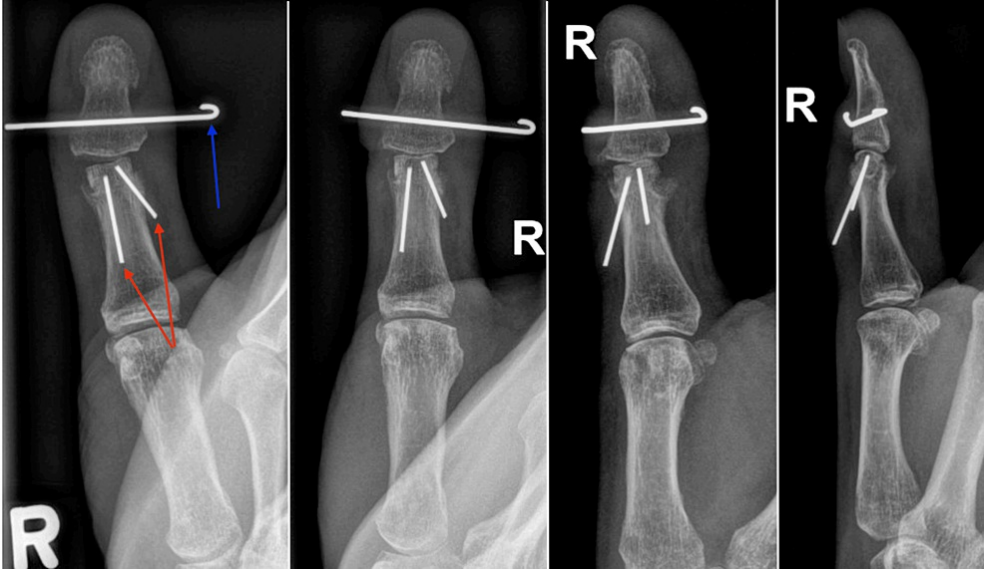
One-month post-operative radiographs showing two retrograde Kirschner wires securing the bone graft (red arrows) and transverse pin through the distal phalanx for static traction (blue arrow).
